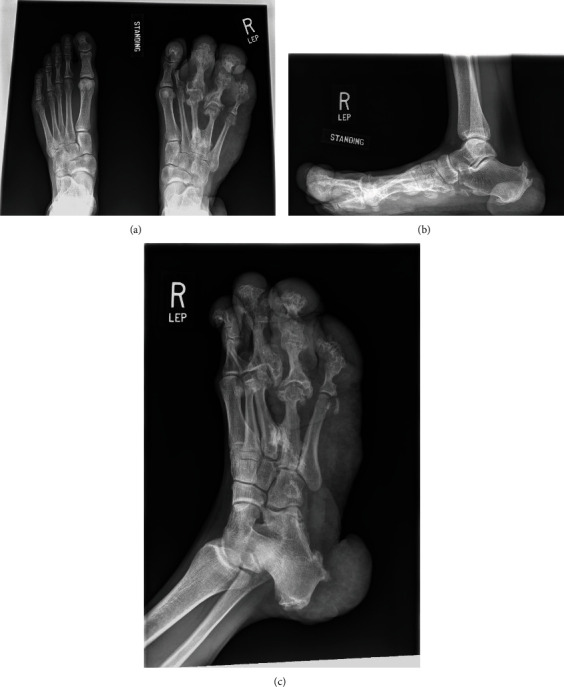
Anteroposterior, lateral, and oblique radiographs of the bilateral feet demonstrating macrodactyly of the right foot involving the second, third, fourth, and fifth toes with medial deviation of the second ray, pseudoarticulation of the third and fourth metatarsals, and advanced degenerative changes of the third and fourth metatarsophalangeal joints and interphalangeal joints of the second through fifth toes. Lateral view demonstrates soft tissue enlargement of the calcaneal soft tissues and large plantar and posterior calcaneal enthesophytes.
